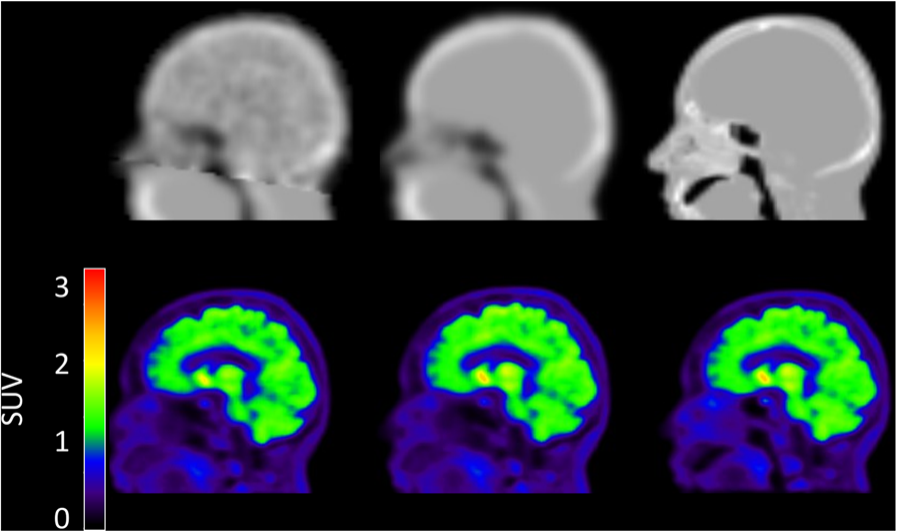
First row, left to right—^68^Ge-, Atlas- and ZTE-attenuation correction (AC) maps; Second row—^11^C-PE2I PET images based on corresponding AC maps expressed as standardised uptake values (SUV)
